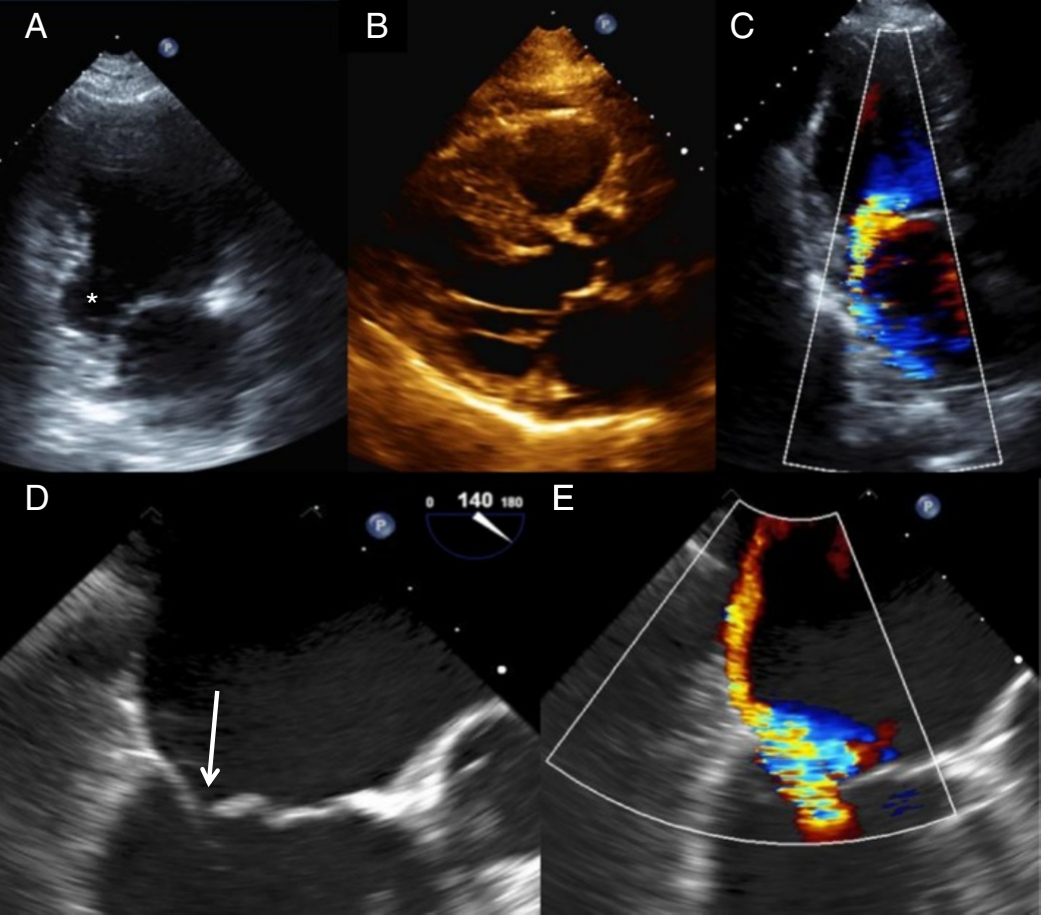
Echocardiographic images of asymmetric CIMR due to inferoposterior myocardial infarct and posterior leaflet tethering. These echocardiographic images were obtained from an 81 year-old male with a history of inferior and inferoposterior myocardial infarction with localized aneurysmal ventricular deformity and atrial fibrillation, when he was evaluated for dyspnea and congestive heart failure. The previous echocardiogram obtained three years prior had demonstrated mild posteriorly directed MR. The apical two chamber view at end-diastole from the current transthoracic echocardiogram shows the true inferobasal aneurysm indicated by an asterisk (A). Asymmetric mitral valve leaflet tenting is depicted in the parasternal long-axis view at mid-systole (B). Additional quantitative measures of tethering phenotype are described in the subsequent main text. The tenting height measured from the mitral annulus plane was 1.4 cm and the tenting area bounded by the mitral annular plane and leaflets was 4.0 cm^2^; tethering angles β and α measured approximately 55° and 40° respectively. The jet of MR was posteriorly directed and reported moderate in severity (C). TEE was then undertaken to confirm the mechanism of MR and this also revealed incomplete mitral valve closure due to PM displacement (D: mid-esophageal long-axis view at omniplane angle 140°, image taken at mid-systole) with pseudoprolapse (arrow) of the anterior leaflet tip relative to the more adversely tethered posterior leaflet. This locus of malcoaptation is the area from which the MR originates. There is severe MR with an eccentrically directed posterior jet (E: mid-esophageal long-axis view at omniplane angle 140°, image taken at mid-systole) with evidence of systolic flow reversal in pulmonary veins (not shown).
